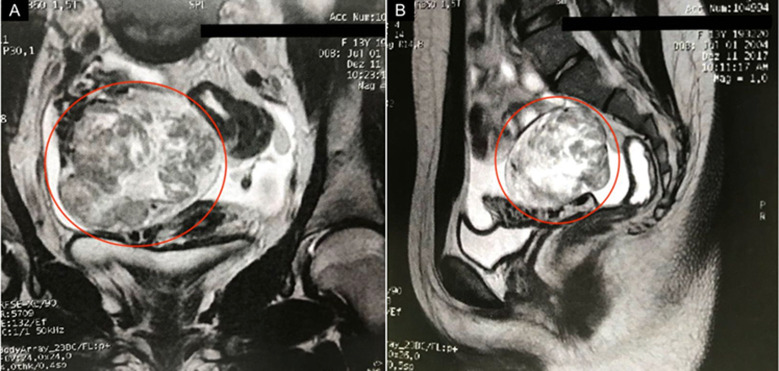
A, B) magnetic resonance imaging of the pelvis (case 1); the circled areas highlight the tumor region
